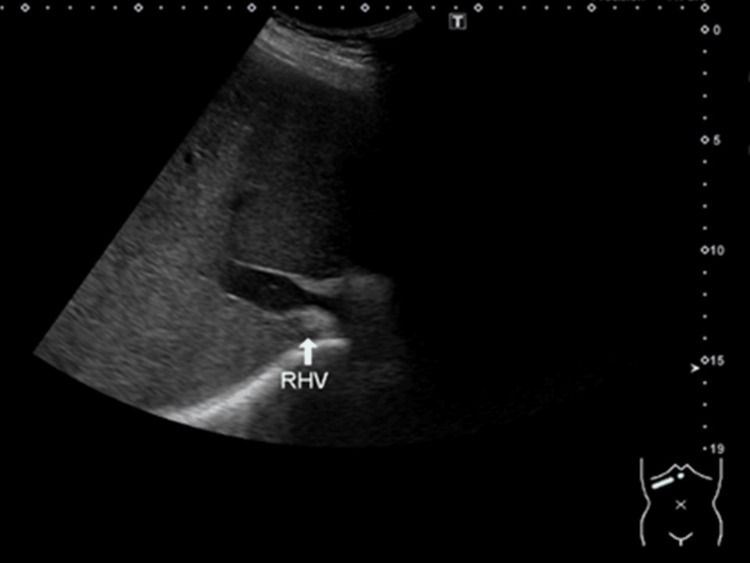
The abdominal ultrasound image demonstrates stenosis of the right hepatic vein (RHV), indicated by the arrow.
